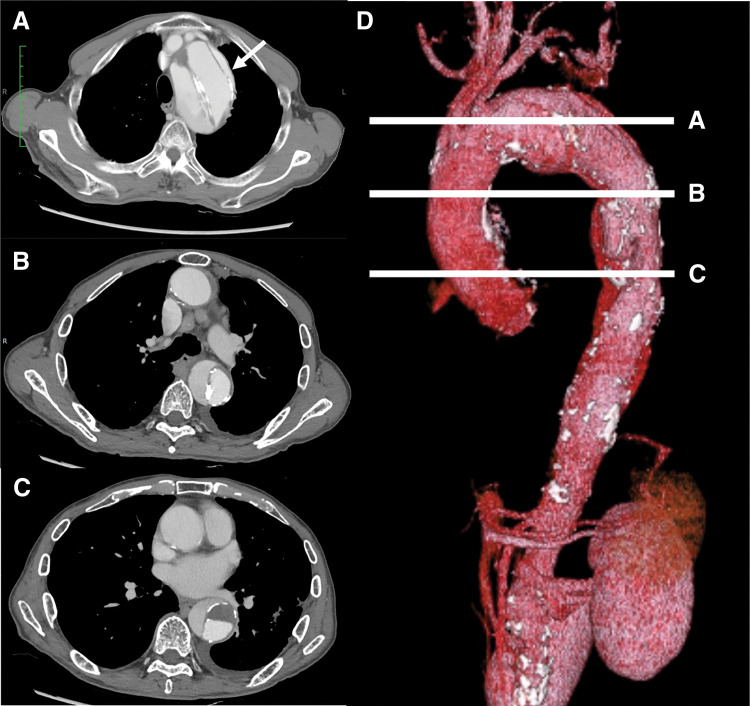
Preoperative CT of aortic arch and proximal descending aneurysm. CT axial images at the levels of the aortic arch and proximal descending aorta, bronchial bifurcation, and left atrium before surgery (panels A, B, and C, respectively). A 3D CT reconstruction before surgery is shown in panel D. White lines A, B, and C in panel D correspond to the axial images in panels A, B, and C, respectively.
